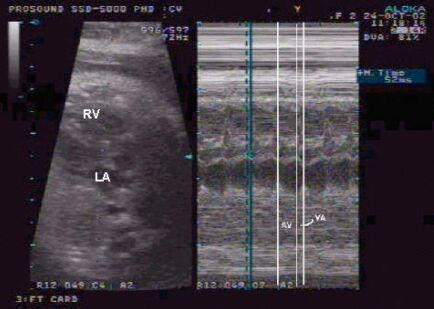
M-mode echocardiography of a SVT with a short VA interval. First white line placed on the peak excursion of the left atrium wall, second line placed on the peak excursion of the right ventricle wall, third line on the consecutive peak atrial wall excursion. The AV interval is markedly longer than the VA interval.
